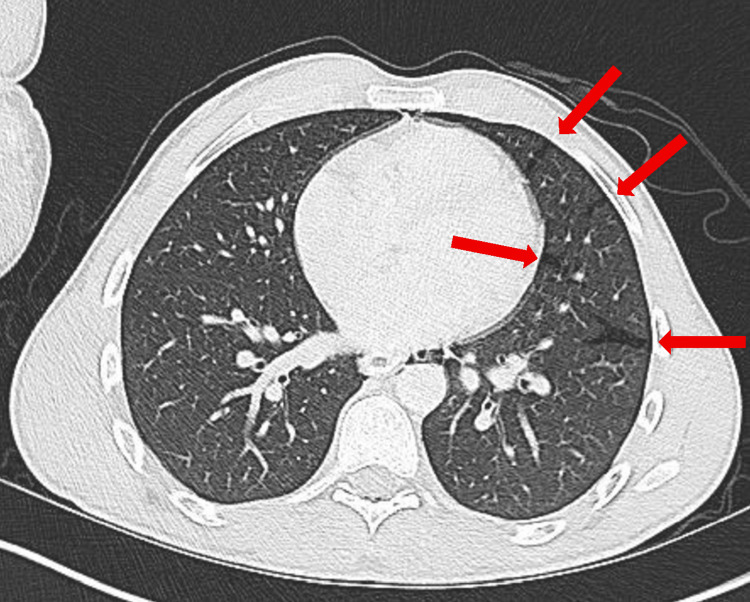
Computed Tomography (CT) scan of the chest (axial view) demonstrating pulmonary lacerations (red arrows).
